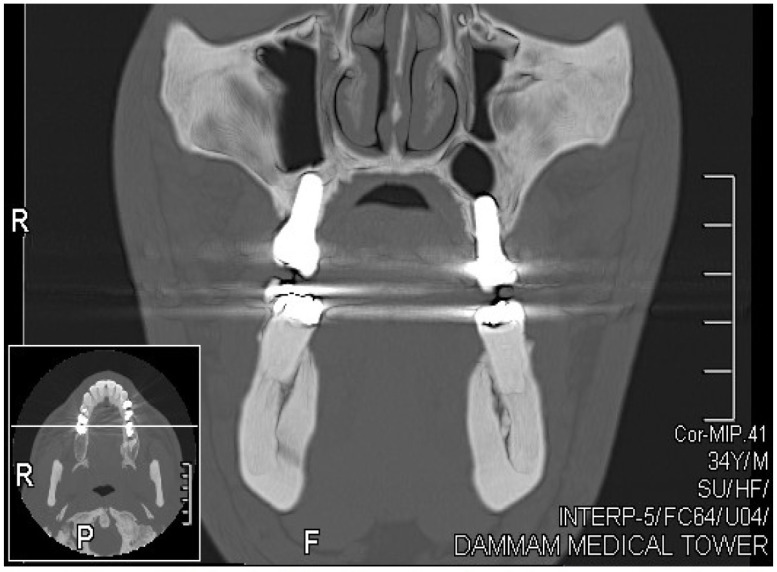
Coronal Computed Tomography showing bilateral bone formation, lateral approach in the right side and crestal approach in the left side.
